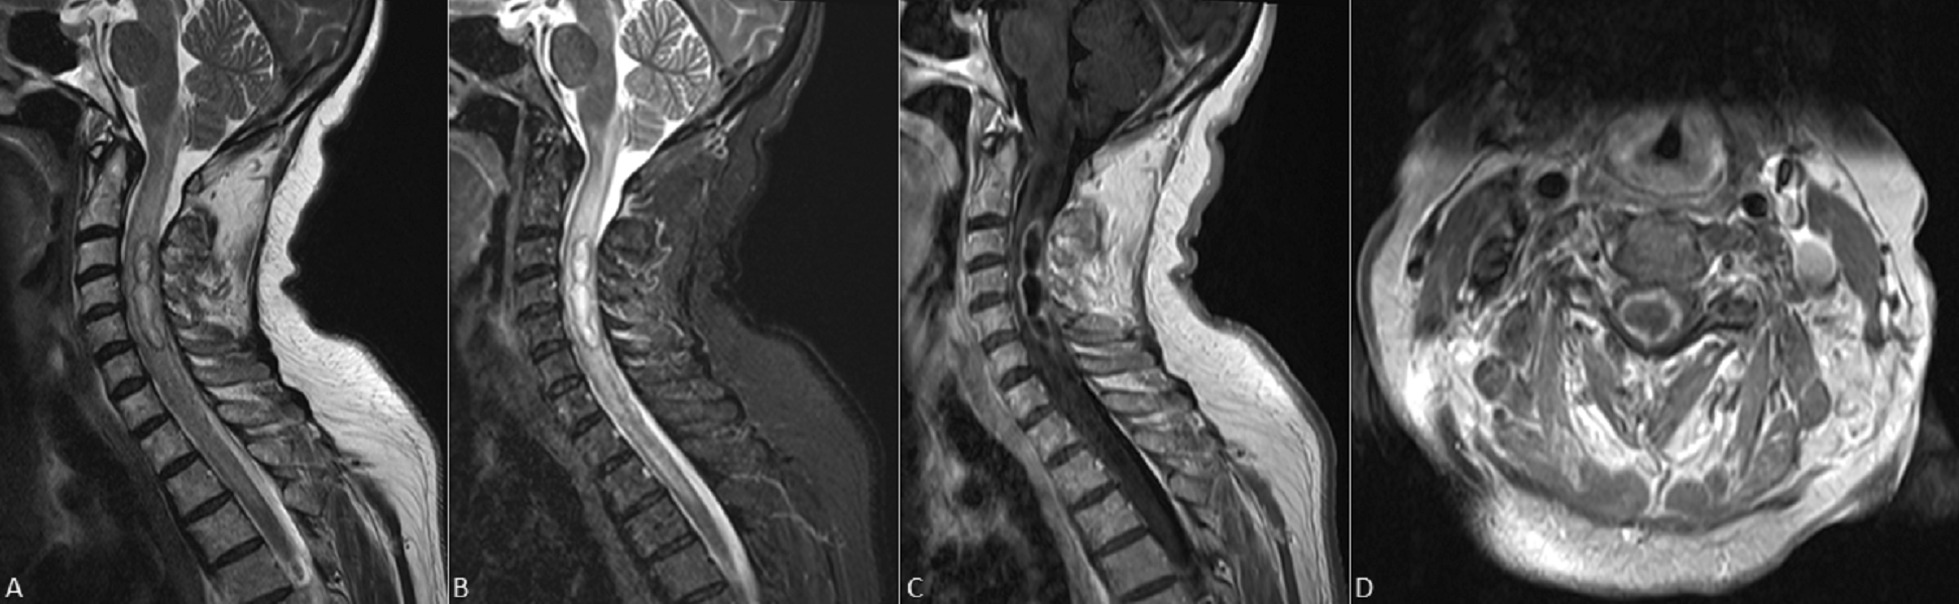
A Sagittal T2-weighted and (B) short tau inversion recovered cervical M.R. images with an intramedullary hyperintense lesion causing diffuse core expansion and edema. C Sagittal and (D) axial T1 post-contrast images demonstrate continuous peripheral ring enhancement
